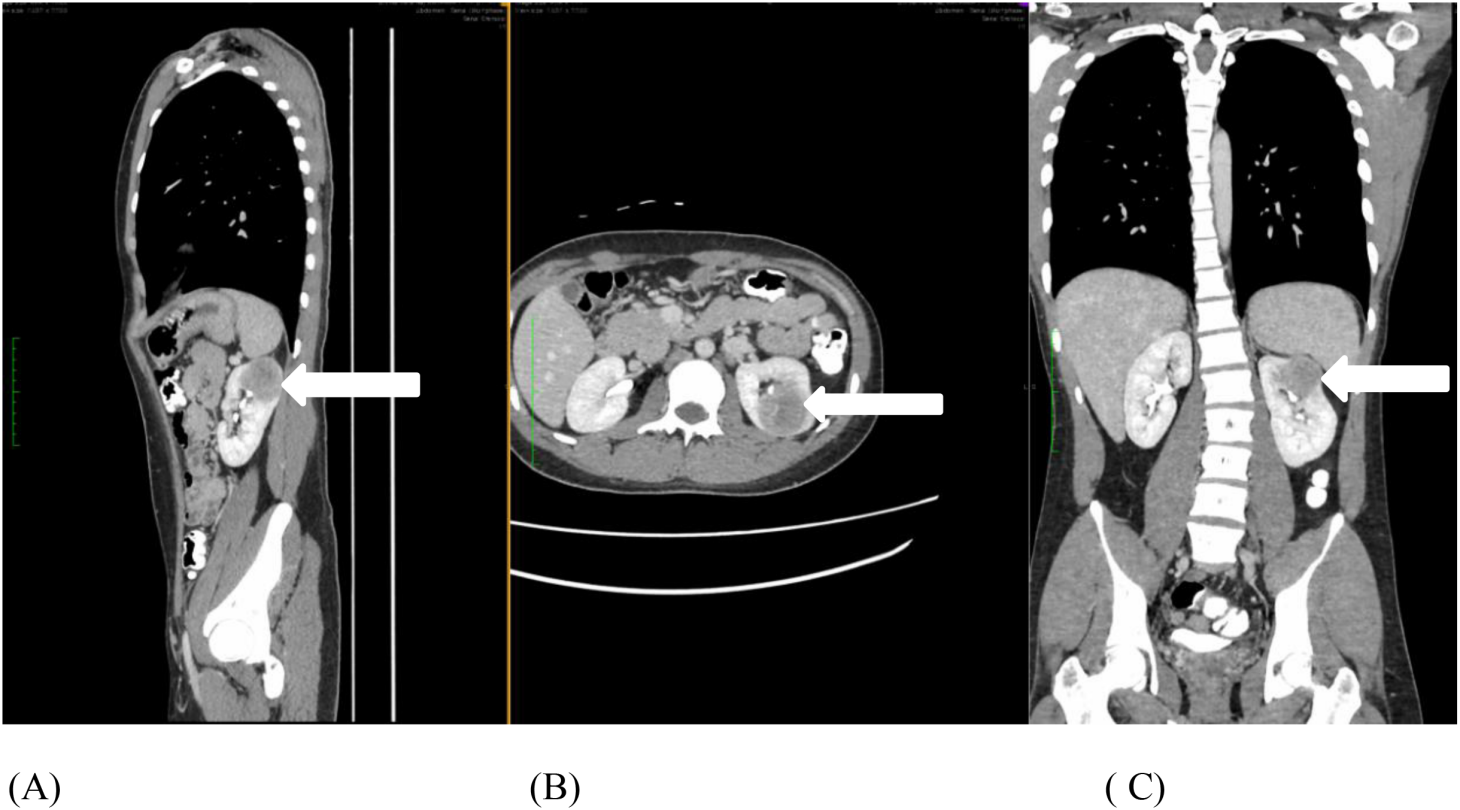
(A-C) Contrast-enhanced computed tomography of the abdomen and pelvis shows an enhanced, ill-defined heterogenous, exophytic lesion with central necrosis at the left upper pole of the kidney measuring 4.7 × 4.1 × 4.2 cm, with perinephric extension (white arrow).
